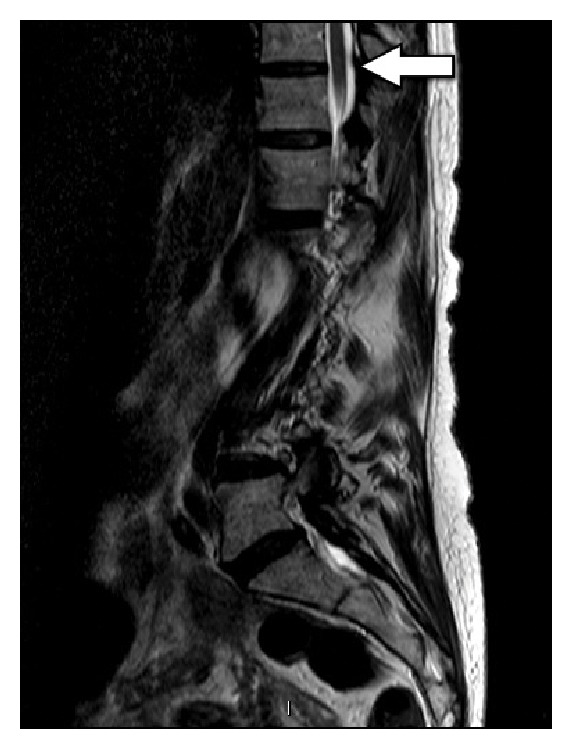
Preoperative sagittal T2 Magnetic Resonance Image. Note the absence of stenosis in the midline of the lower thoracic spine. The arrow points to the T10-11 level.
